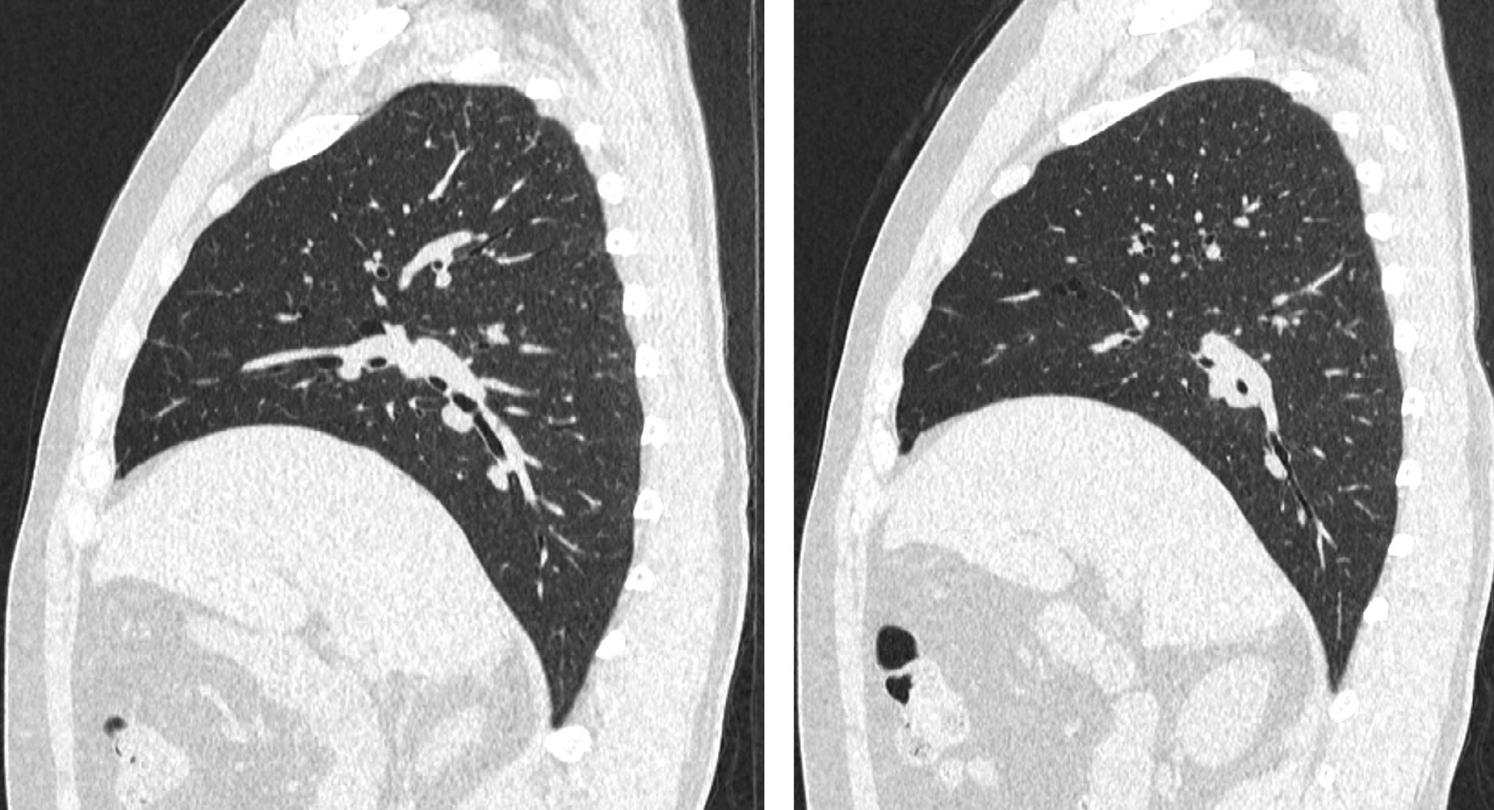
CT Chest without contrast: Sagittal images demonstrate multiple thin-walled elliptical well-defined para-mediastinal air-filled cysts in a basilar distribution with preserved lung volume without evidence of interstitial lung disease
